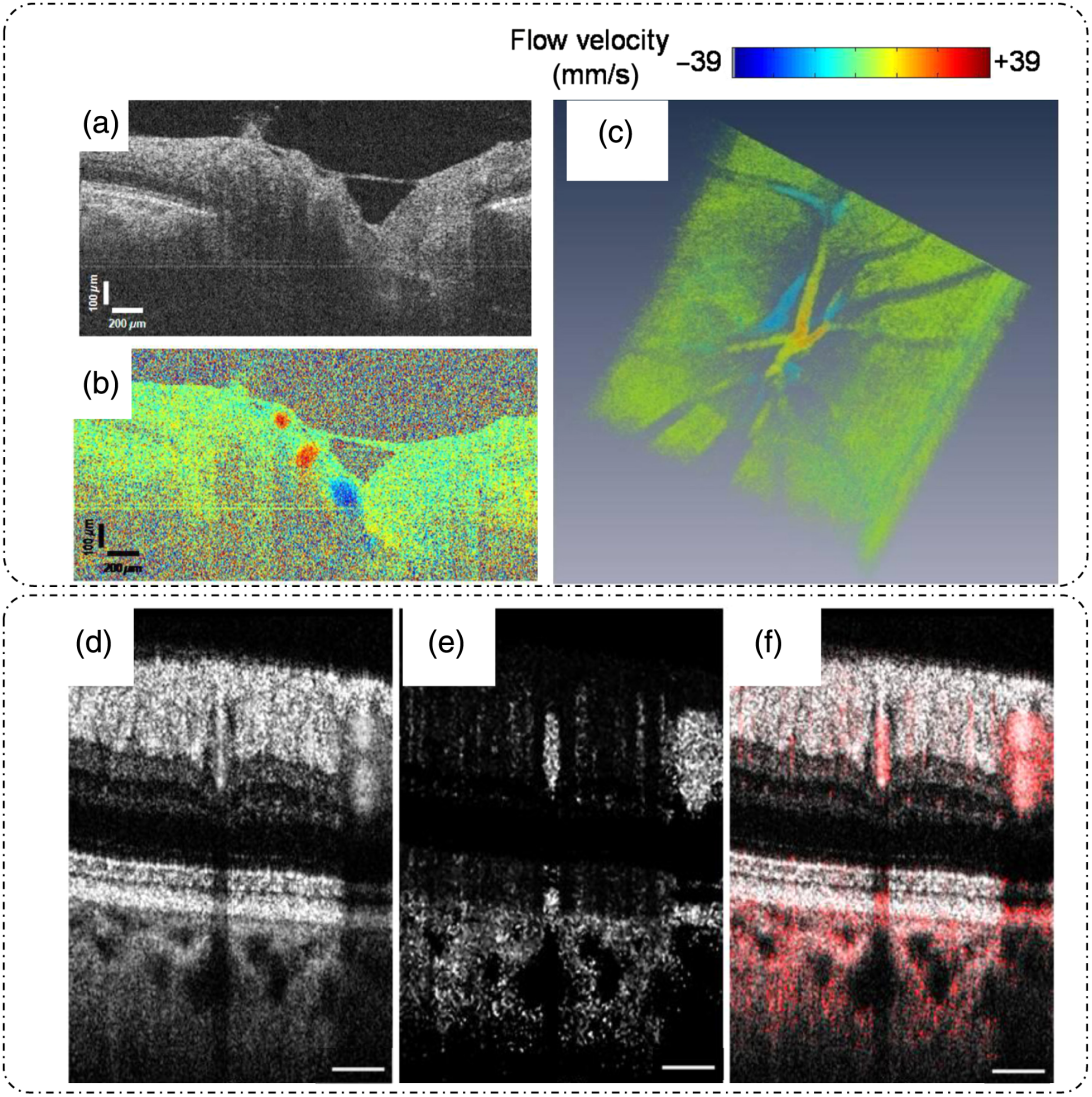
Applications of Doppler OCT and pvOCT. Doppler OCT: (a) retinal structural reflectivity B-scan, (b) Doppler OCT image at 200 kHz, and (c) 3D tomogram of Doppler OCT images at 200 kHz. Phase-variance OCT: (d) retinal OCT average intensity image, (e) phase-variance image, and (f) overlay map with average intensity in gray and phase variance in red. Scale bars for panels (d)–(f) are 250  μm. (a)–(c) Reproduced with permission from Ref. 20, © 2011, Optica; (d)–(f) reproduced with permission from Ref. 70, © 2011, Optica.
